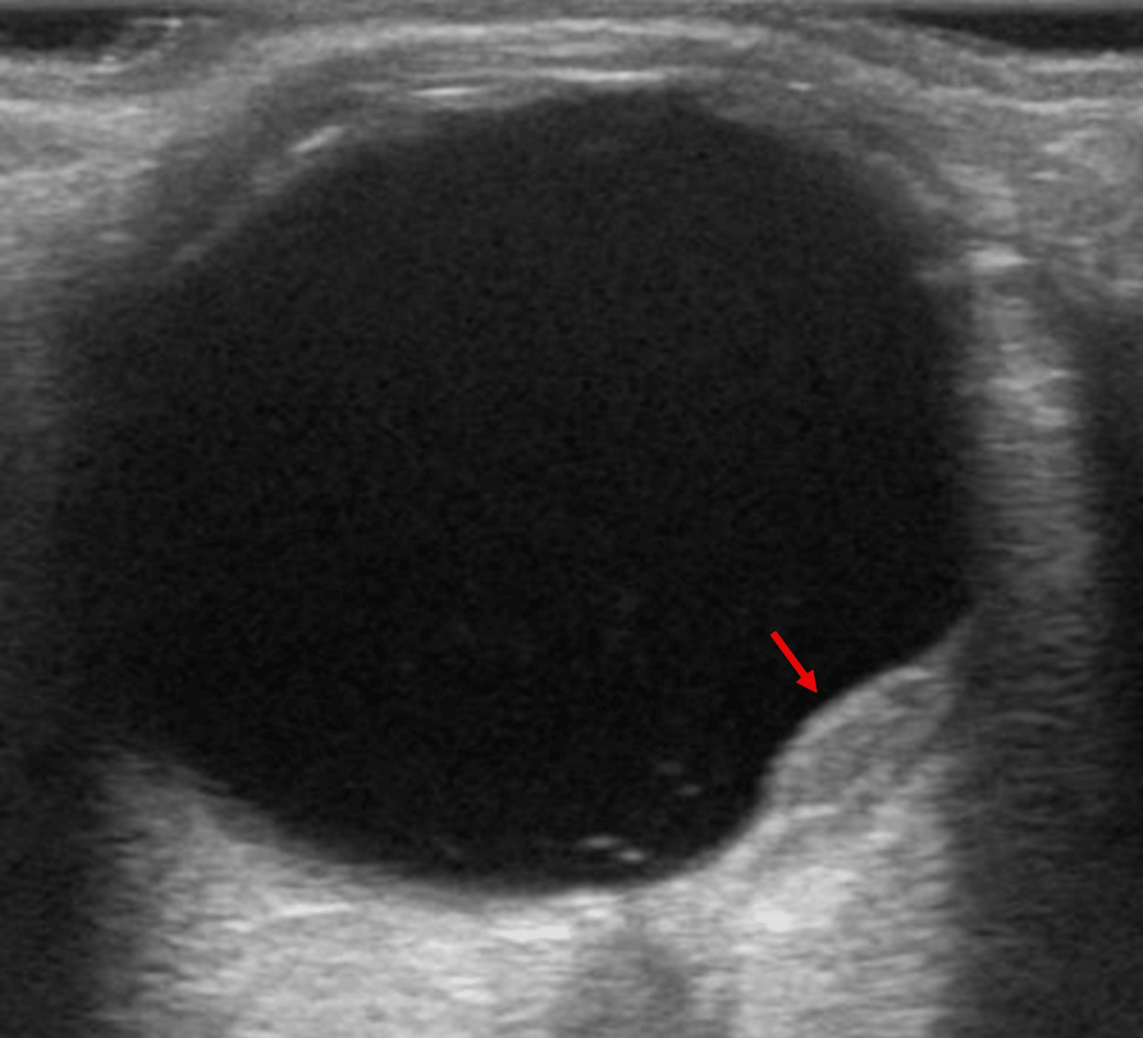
Ultrasonography of the left eye Ultrasonography of the left eye also shows the choroidal mass lesion (red arrow).
